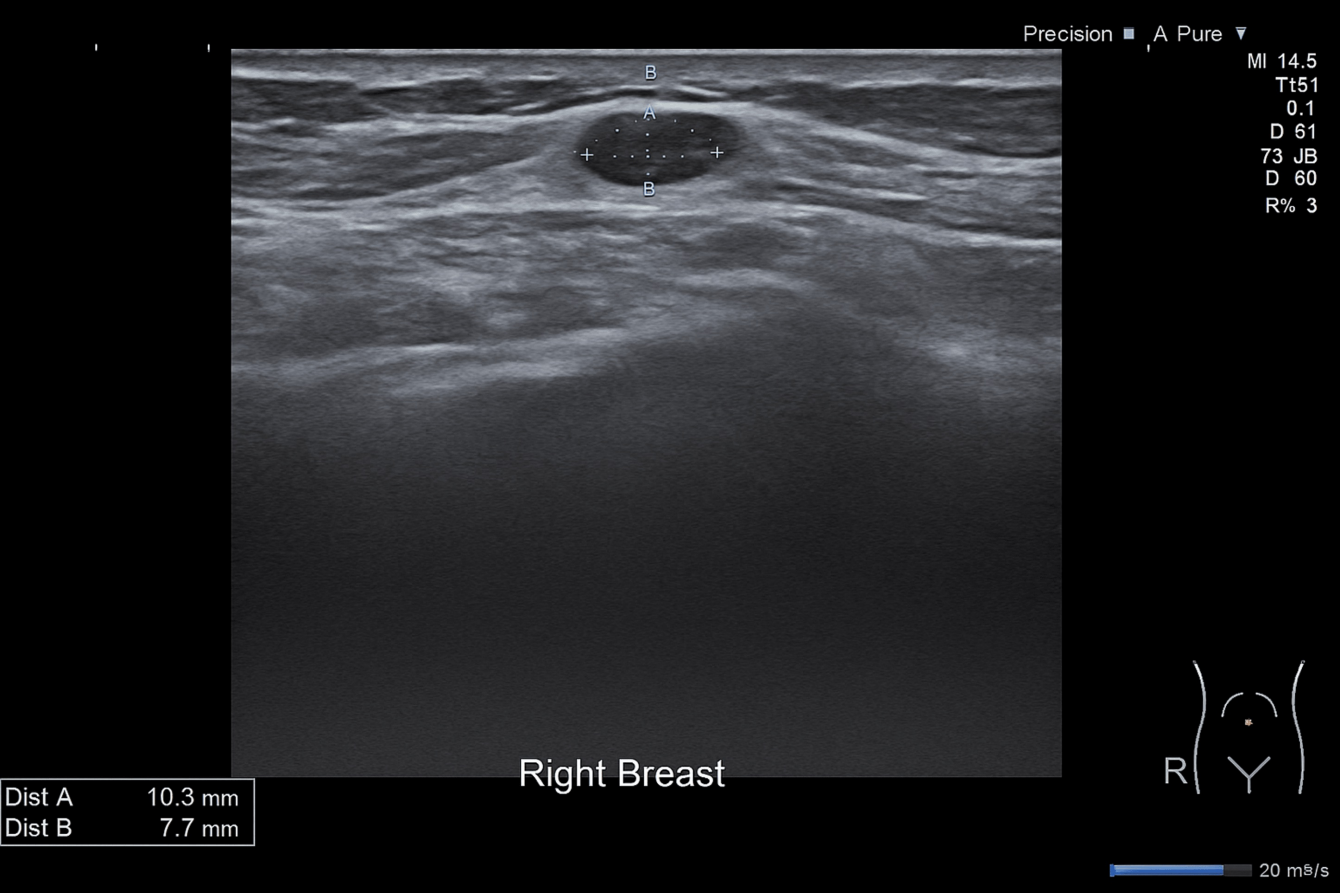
Ultrasound scan of the right breast showed a 10mm ill-defined mass in the upper inner quadrant.
